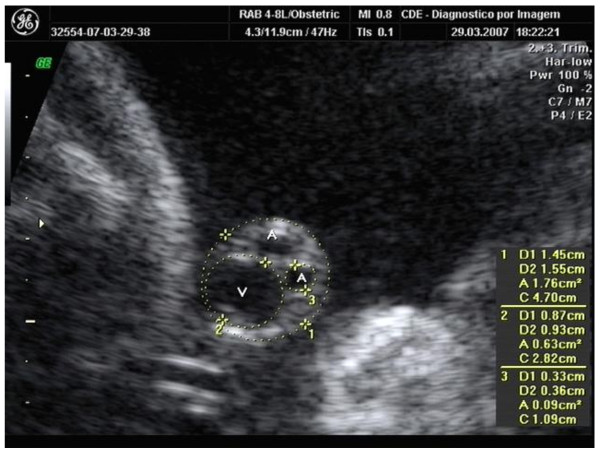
Ultrasonographic measurement of the cross-sectional area of the umbilical cord (C), of the diameter of the umbilical vein (V) and of the umbilical artery (A). The area of Wharton Jelly (WJ) is WJ = C-V-2A.
